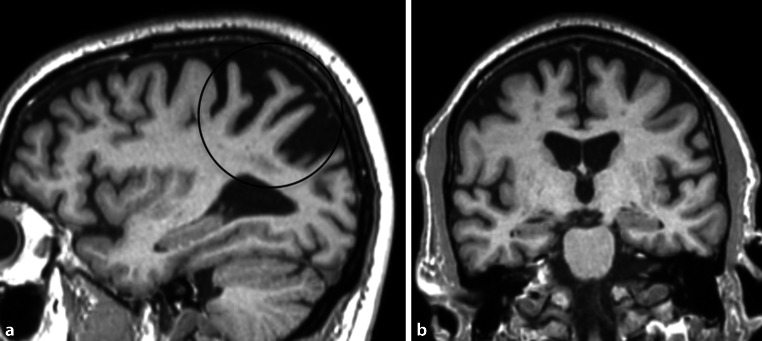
Atypical Alzheimer’s disease. A 58-year-old female patient with early-onset Alzheimer’s disease who presented with marked posterior cortical atrophy (depicted in a on sagittal T1-weighted images marked with a *circle*), while the hippocampi were relatively well preserved (shown in b on coronal T1-weighted images)
